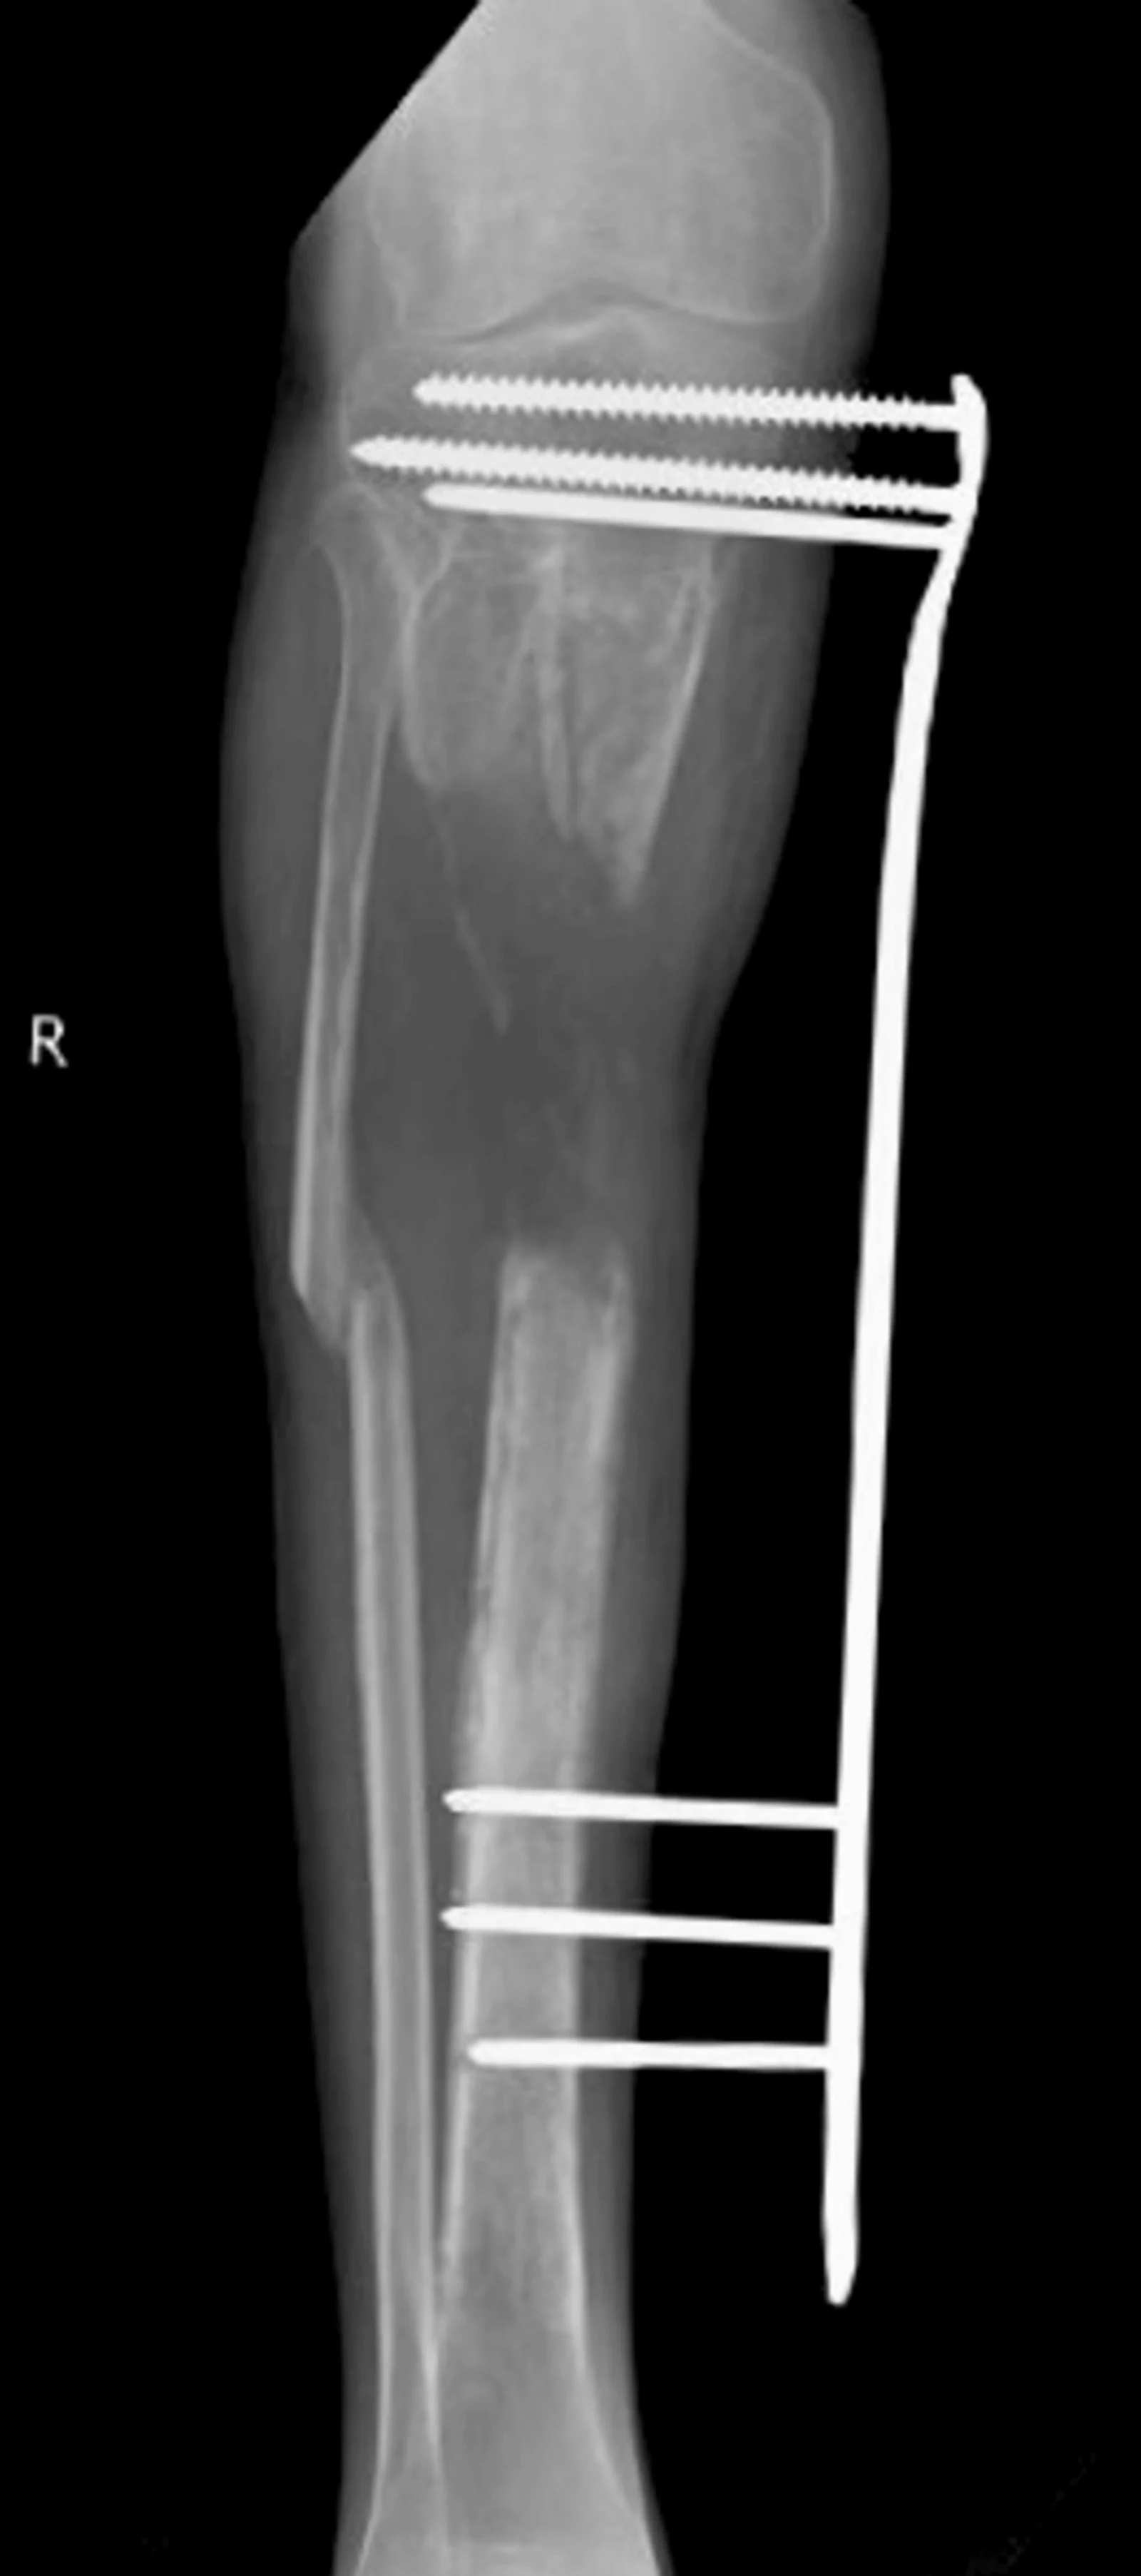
Pre-operative X-ray of the right leg
